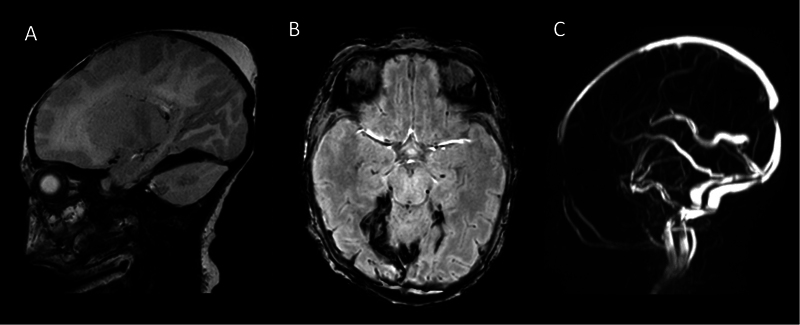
Neuroimaging on admission. MRI, postnatal day 6. (
